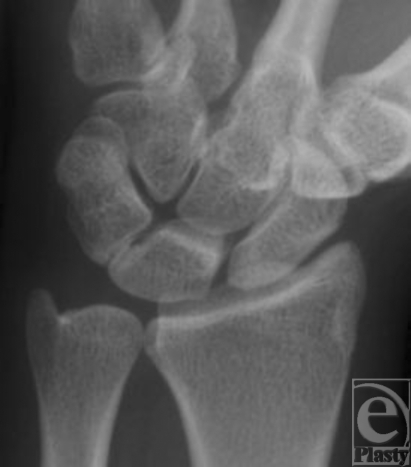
Anteroposterior radiograph of the left asymptomatic wrist showing a luno-triquetral coalition Minaar type I.
